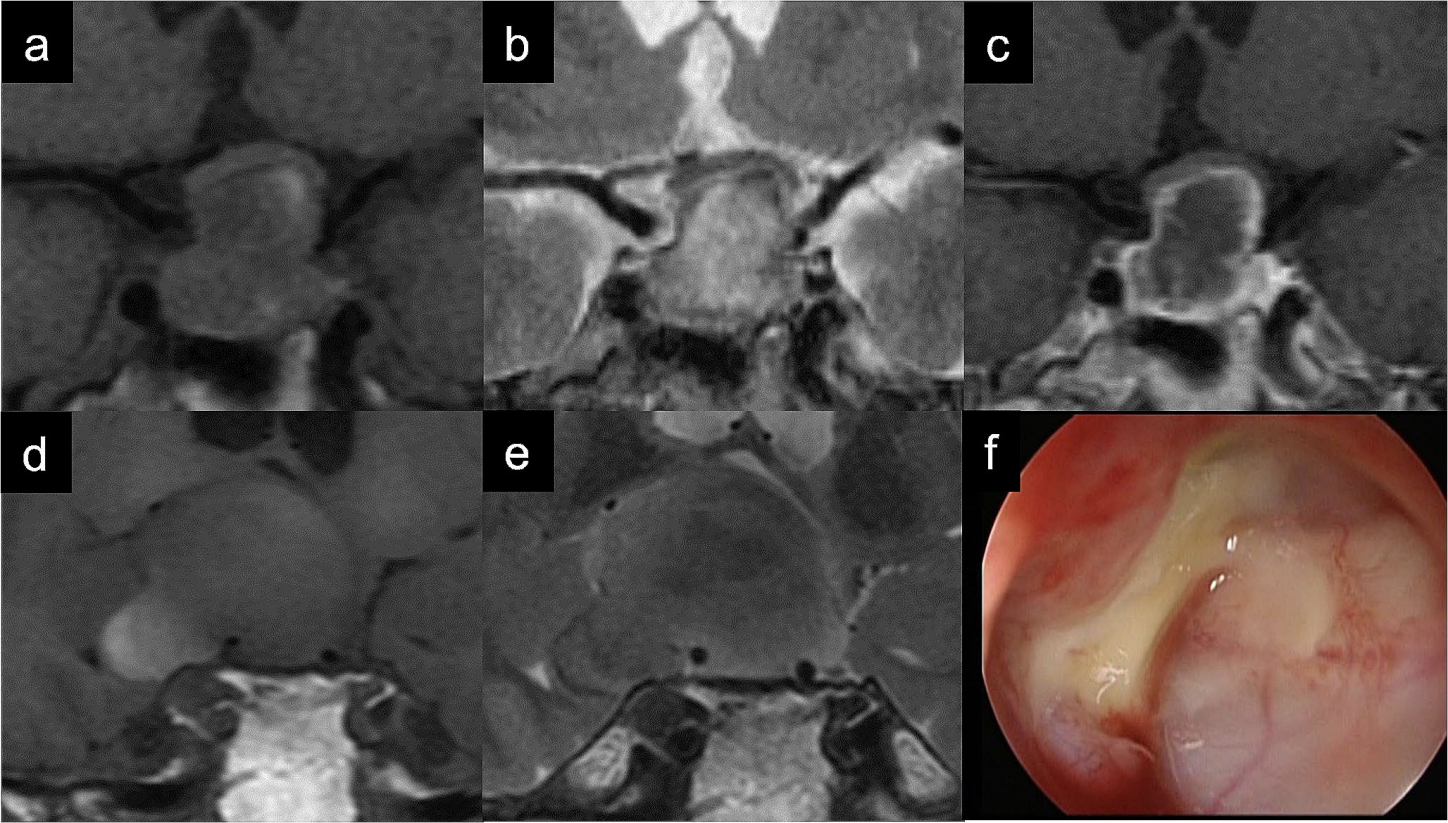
Representative recurrent case for type A Rathke’s cleft cyst (Patient No. 1). (a) T1-weighted image (T1WI) and (b) T2-weighted image (T2WI) showing mixed low and high signal intensity in the cyst; (c) Contrast-enhanced T1WI showing cyst wall enhancement; (d) T1WI obtained at the time of cyst recurrence showing high signal intensity in the lateral part of the cyst; (e) T2WI obtained at the time of recurrence showing mixed low and high signal intensity in the cyst; (f) Intraoperative findings at recurrence showing purulent contents on the lateral side of the cyst
